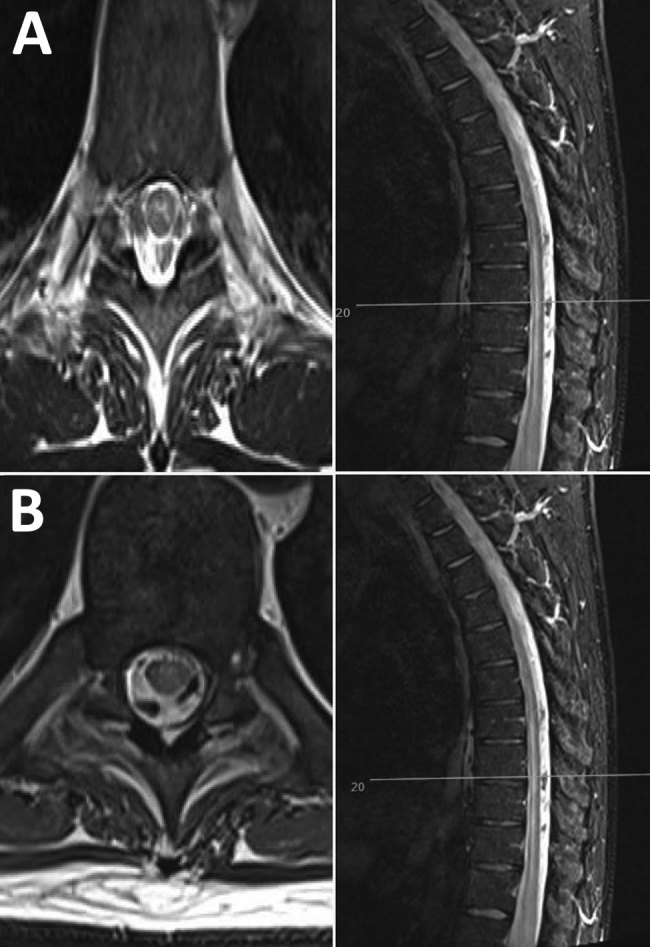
Neurologic manifestations of tick-borne encephalitis in a 38-year-old man from the United Kingdom after travel to Lithuania. A) Magnetic resonance imaging of the brain and spinal cord at onset of neurologic signs, showing possible longitudinal extensive transverse myelitis in the cervical and thoracic cord, with involvement of the central gray matter. B) One month later, increased T2 signal and mild swelling of the central gray matter of the cervical cord have both regressed, with some residual subtle signal changes throughout the spinal cord. Left, axial images; right, sagittal images.
